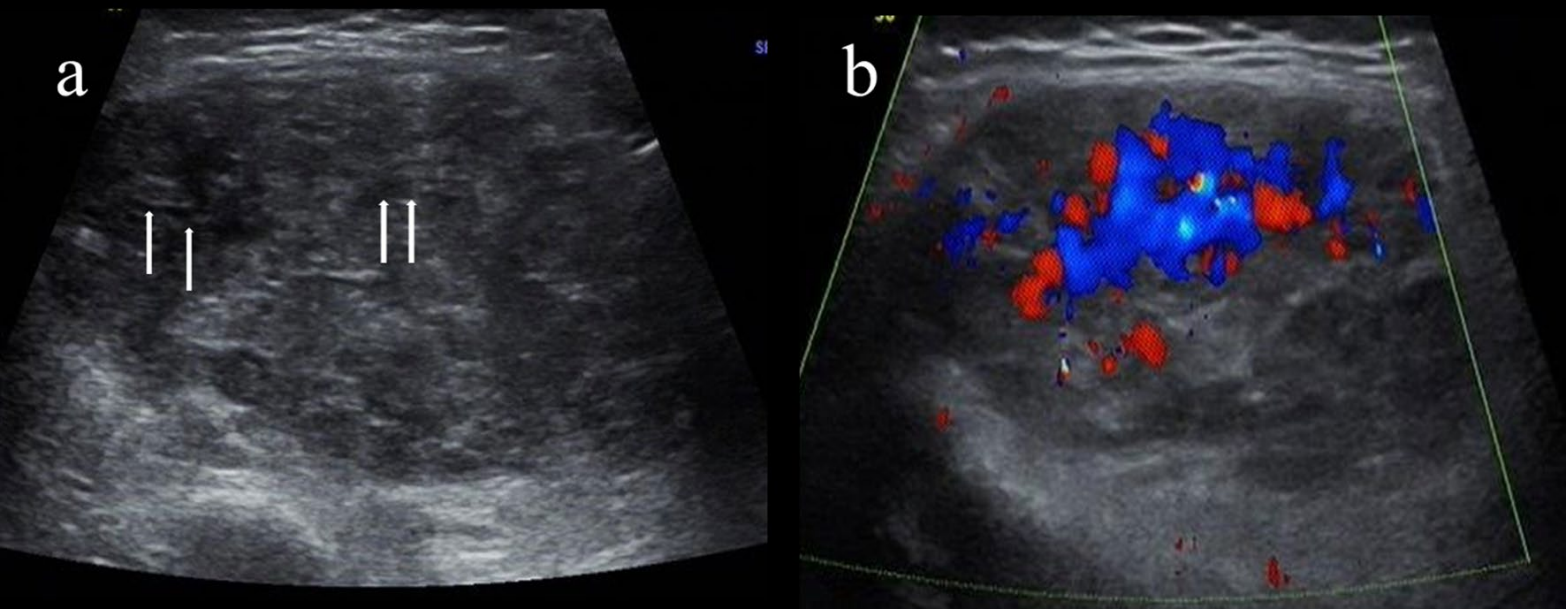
In a 2-year-old girl with claudication for a week, a mass was found in her right knee, which was later diagnosed as hemangioma. (a) Gray-scale US shows an ill-defined mass in the right knee with a maximum diameter of 52 mm. Linear anechoic spaces (arrows) are consistent with slow-flow venous channels. (b) Color doppler flow imaging shows rich central hyperemia.
